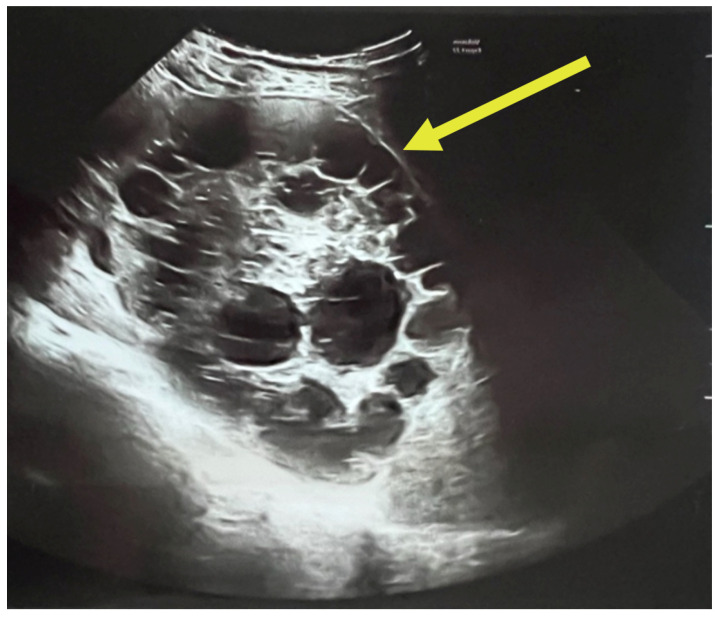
Ultrasound of the hydatid cyst with internal septations and vesicles: numerous daughter vesicles of varying sizes are visualized, separated by fine septations, enhancing the suspicion of active parasitic proliferation.
